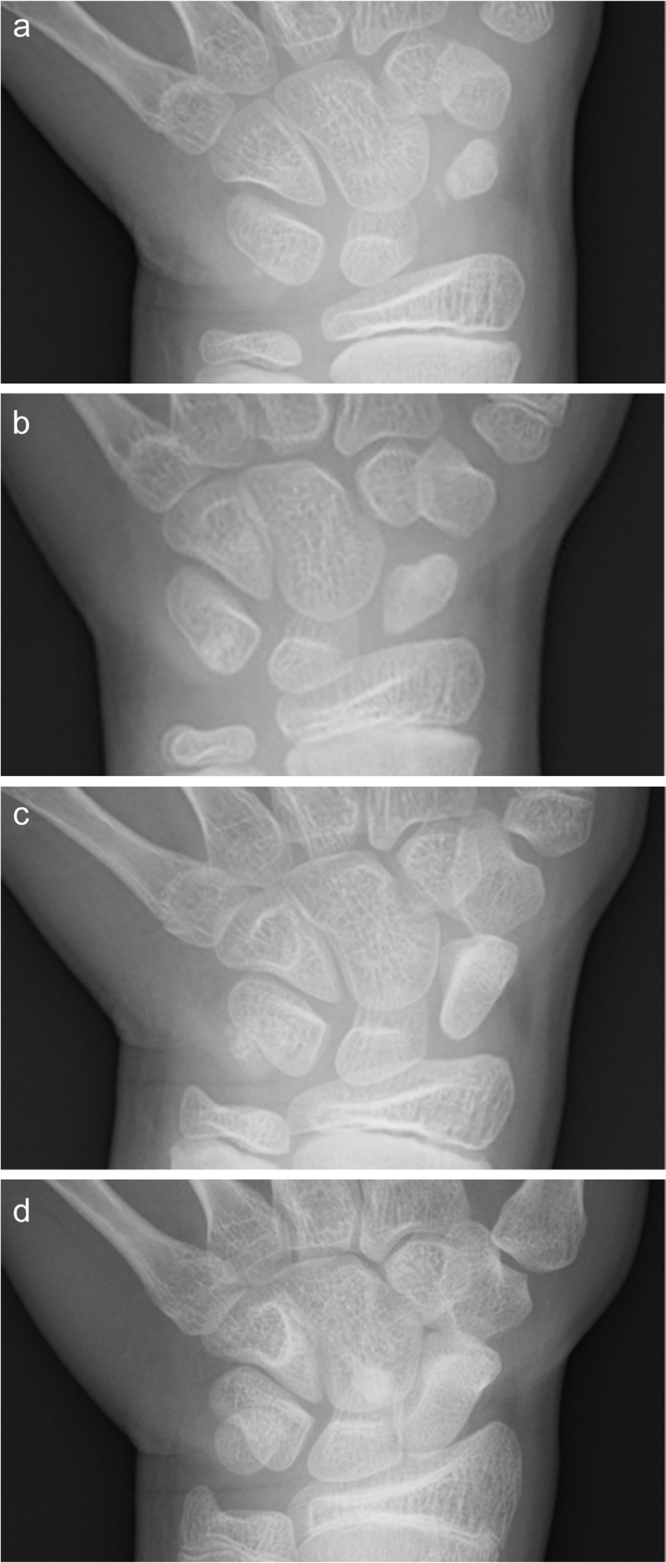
Temporal changes in plain radiography. After 2 month of orthosis immobilisation followed by 3 weeks of immobilisation using a thumb spica cast, the distal pole of the scaphoid showed remodelling (a). At 1 year after the initial visit, plain radiography showed remodelling of the proximal pole (b). At 2 years after the initial visit, the whole scaphoid bone showed remodelling (c). At 5 years after the initial visit, scaphoid bone showed normal growth (d)
